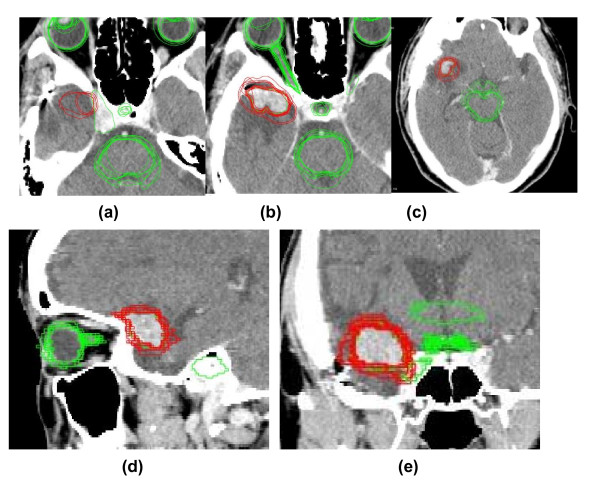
Contours superimposed on the default CT image (Meningioma). (a) (b) (c) axial section (d) sagittal section (e) coronal section The red lines depict planning target volumes, and the green lines depict delineations of organs at risk from each clinician.
